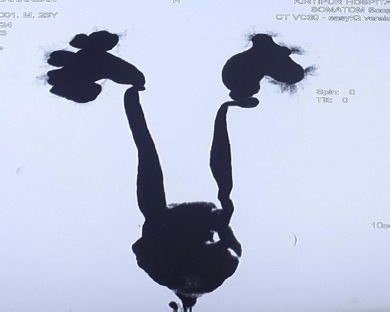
Bilateral moderate hydroureteronephrosis on Computed Tomography Urogram.
